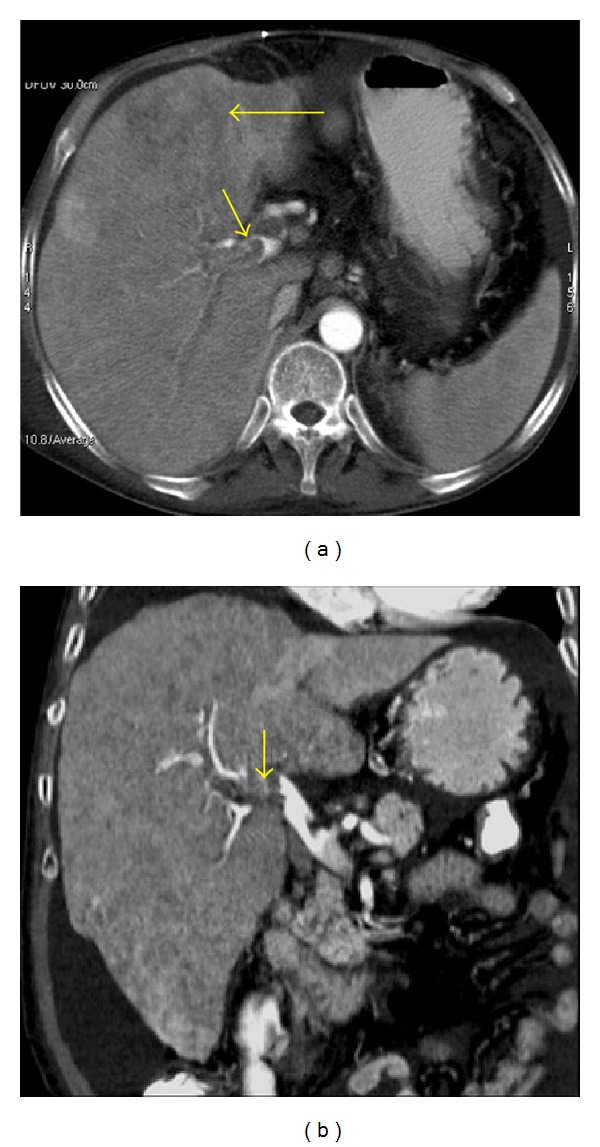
Portal vein thrombosis in a patient with HCC complicating hepatic cirrhosis. (a) Arterial phase contrast enhanced axial CT image shows a large filling defect in the portal vein indicating endoluminal thrombus (short arrow). A small peripheral HCC is noted in the hepatic segment 4 (long arrow). (b) a Coronal MIP reconstruction best depicts the filling defects in the main portal vein (arrow).
